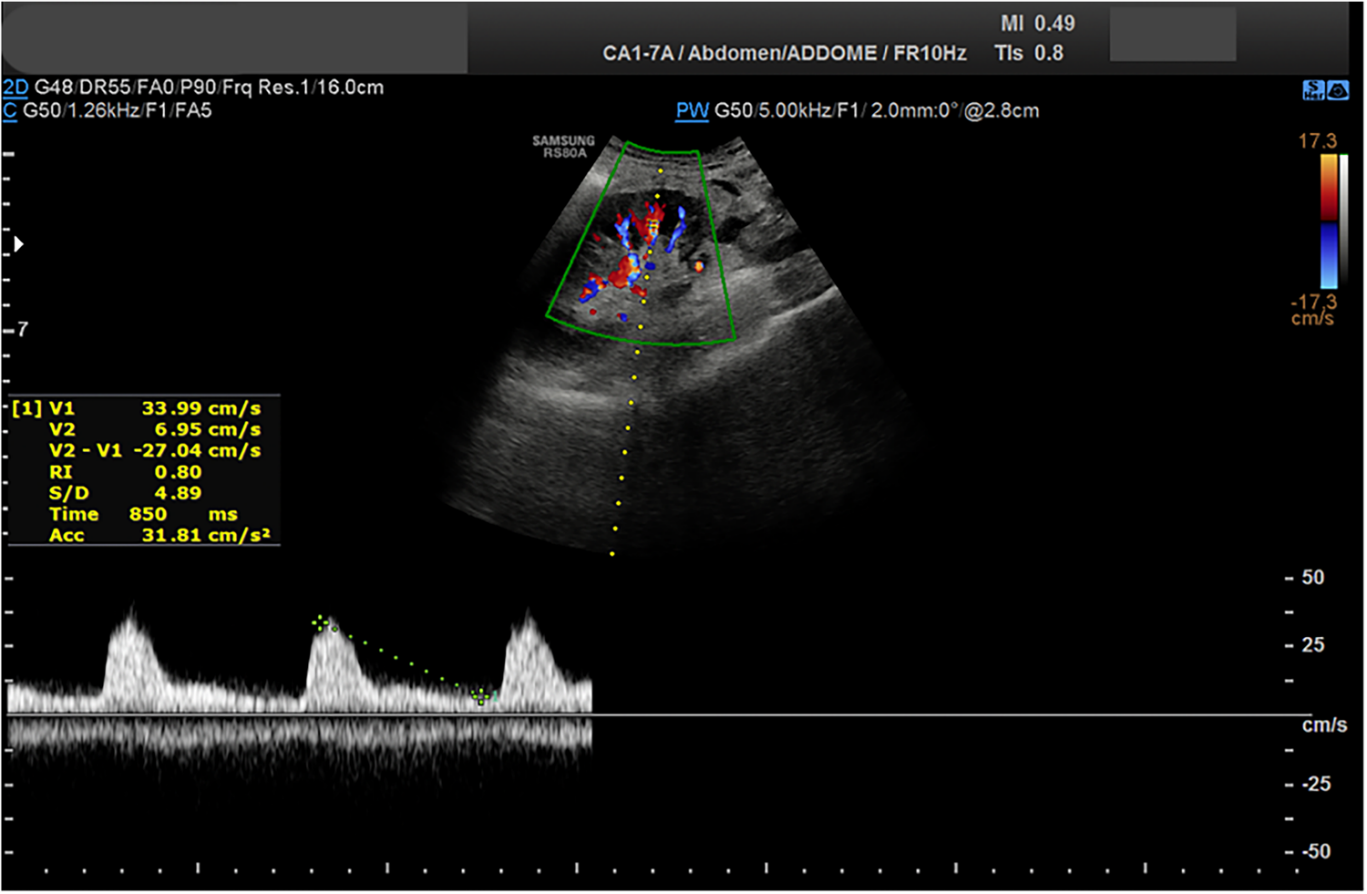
The Color-Doppler shows normal RI at the level of the segmental arteries in a Patient whit renal ischemia after renal transplantation
